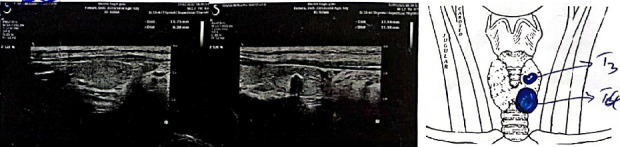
Color Doppler sonography of the thyroid gland and neck. The findings indicated that both thyroid lobes have small size and heterogeneously decreased parenchymal echo suggesting chronic lymphocytic thyroiditis. Two focal lesions at the left lobe were seen: N1: an 18 ∗ 13 ∗ 11.7-mm well-defined isoechoic solid nodule contains coarse and fine calcifies foci and border line elastoscan is seen at lower part (TRIDAS Iva, moderately suspicious). N2: an 11.7 ∗ 6.3 ∗ 7.8-mm well-defined isoechoic without calcification with peripheral vascularity and soft elasticity as elastoscan is seen at the junction of isthmus to the left lobe (TIRADS III, mildly suspicious).
